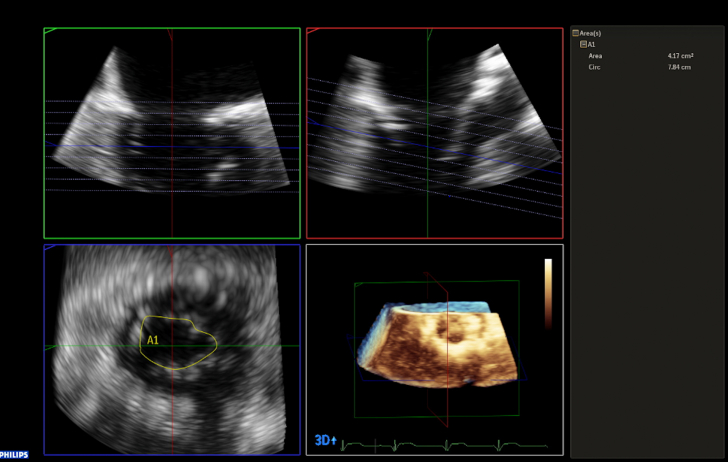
Assessment of Mitral Transcatheter Edge-to-Edge Repair Feasibility (Part 1) Measurement of mitral valve area by three-dimensional multiplanar reconstruction on transesophageal echocardiography.
